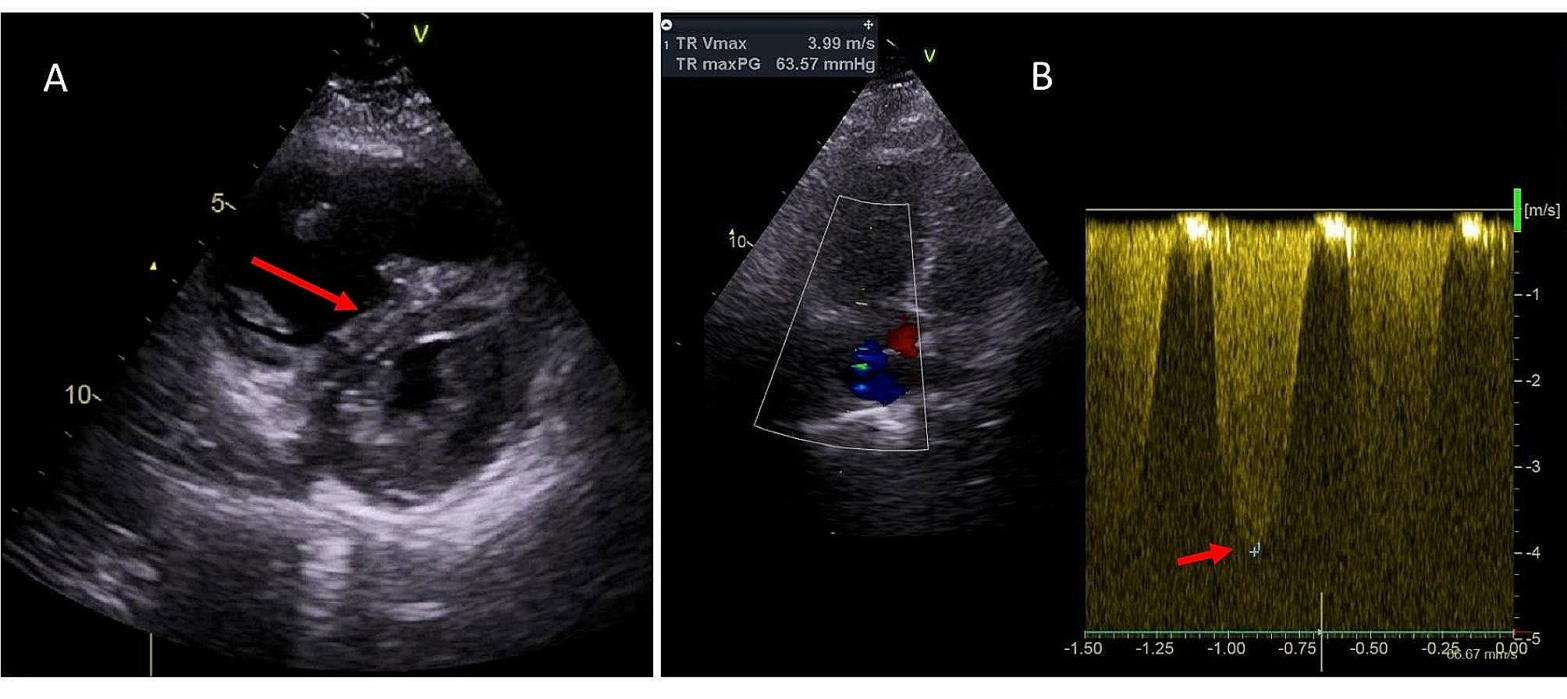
Transthoracic echocardiogram: (A) Parasternal short axis image, showing dilated right ventricle with systolic D-shaping of the interventricular septum (arrow); (B) Continuous wave doppler in apical four chamber view, showing estimated pulmonary artery systolic pressure of 64 mmHg + central venous pressure, as measured by tricuspid regurgitation jet velocity (arrow)
